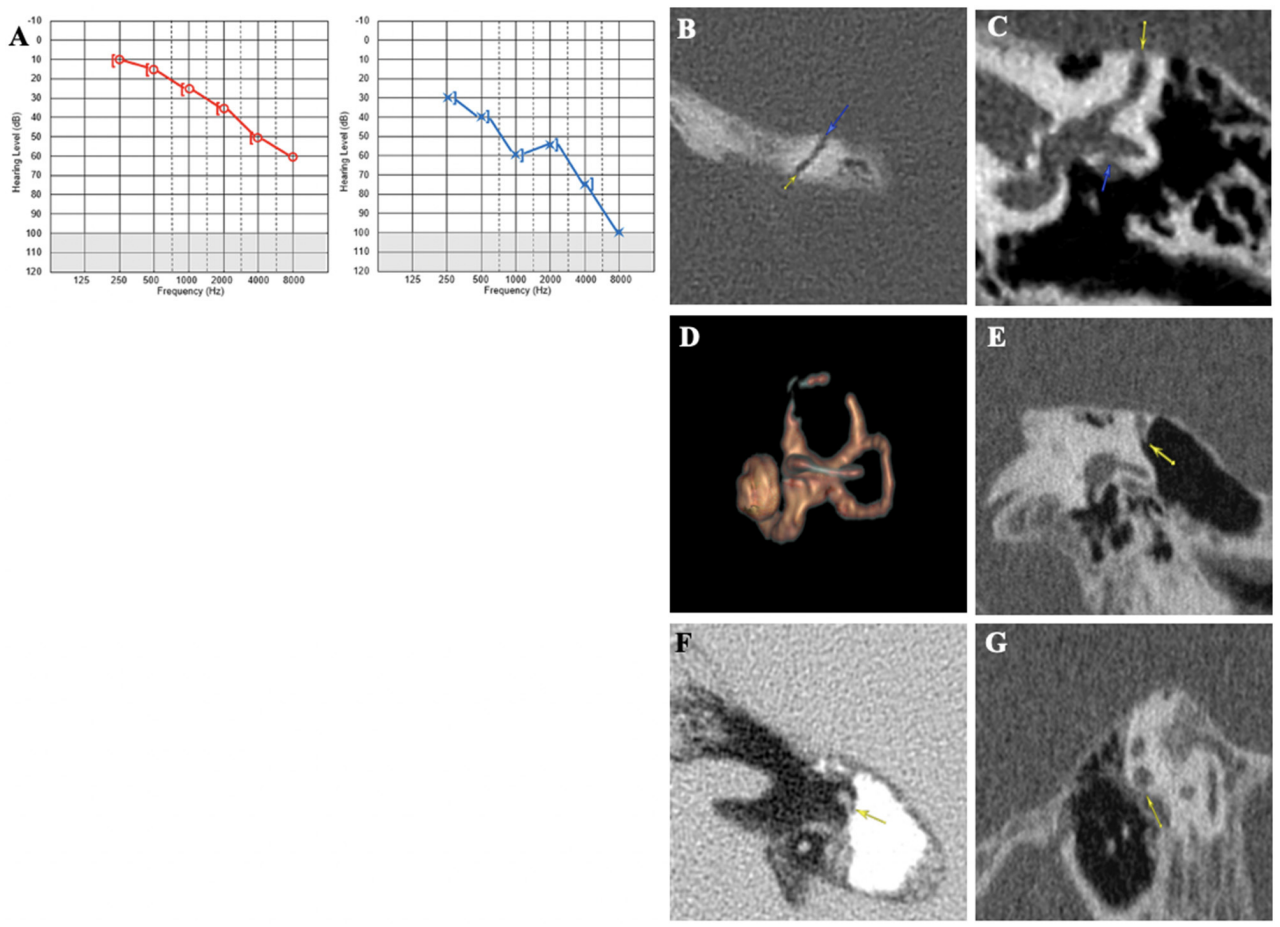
(A) Tonal audiometry showing a bilateral sensorineural hearing loss, more pronounced on the left side. (B, C) Left SSCD in axial (B) and coronal plane (C) and CFD (blue arrow) (C). (D) Post-operative 3D MRI showing a correctly plugged superior canal. (E–G) Post-operative HRCT control showing CFD and another location of SCCD near the surgical approach (yellow arrow).
